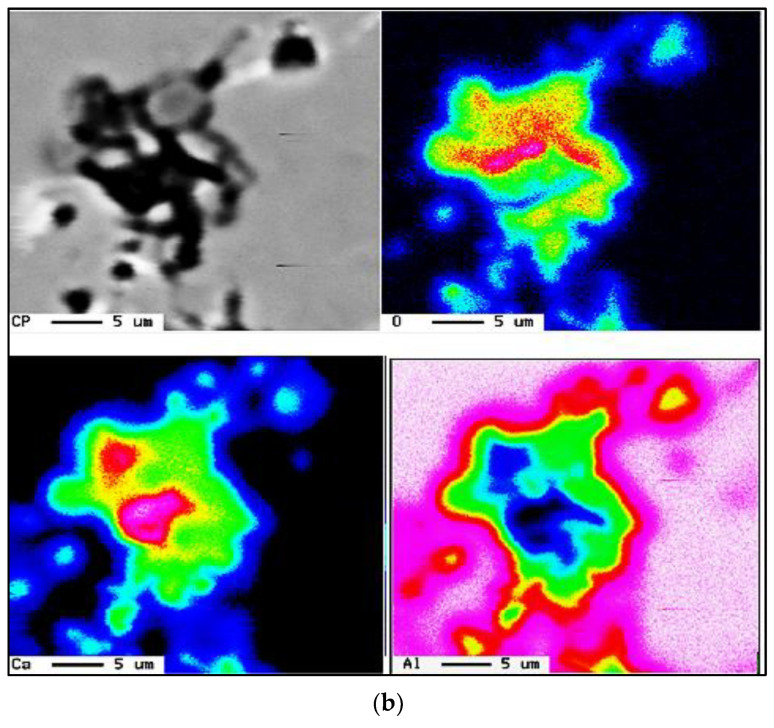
Examples of (a) Bi–Sr–Mg–O interactions, (b) X-ray mapping of Al-Ca-O particles in the captured picture (CP) shown in (b)—see Figure 5f.
